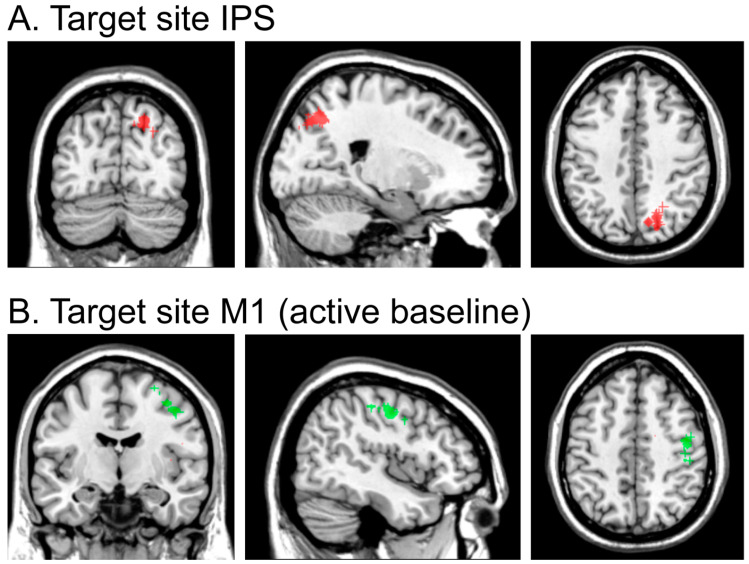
Individual, MRI-guided TMS target sites for all participants. (A) Target site in the IPS (shown in red in the figures) with the associated mean MNI coordinates [20, −69, 44]. (B) Target site M1 (shown in green in the figures) as used in the active baseline condition, with the associated mean MNI coordinates [41, −10, 51].
